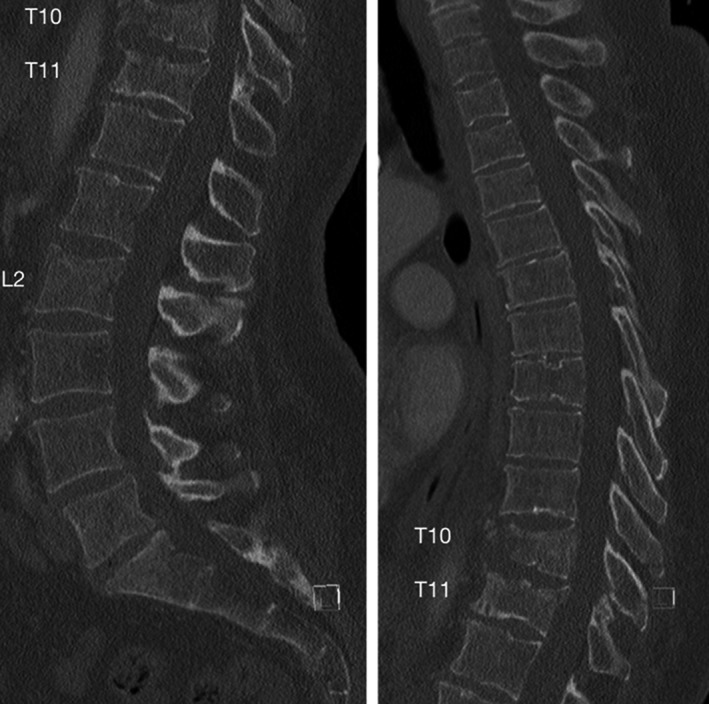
Preoperative CT‐scan demonstrating acute T10, T11, and L2 compression fractures with worse comminution at T10
